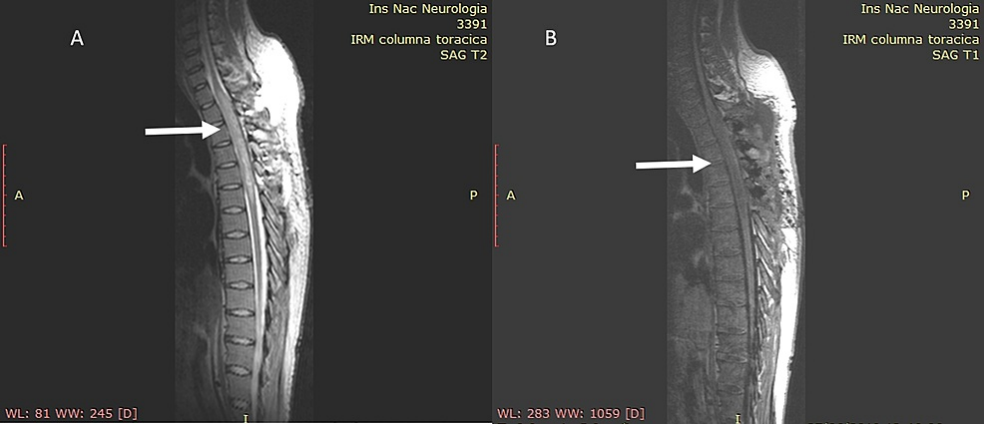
MRI spine, sagittal view showing (arrows) a lesion at T2-T6. A) T2- and B) T1-weighted images.
